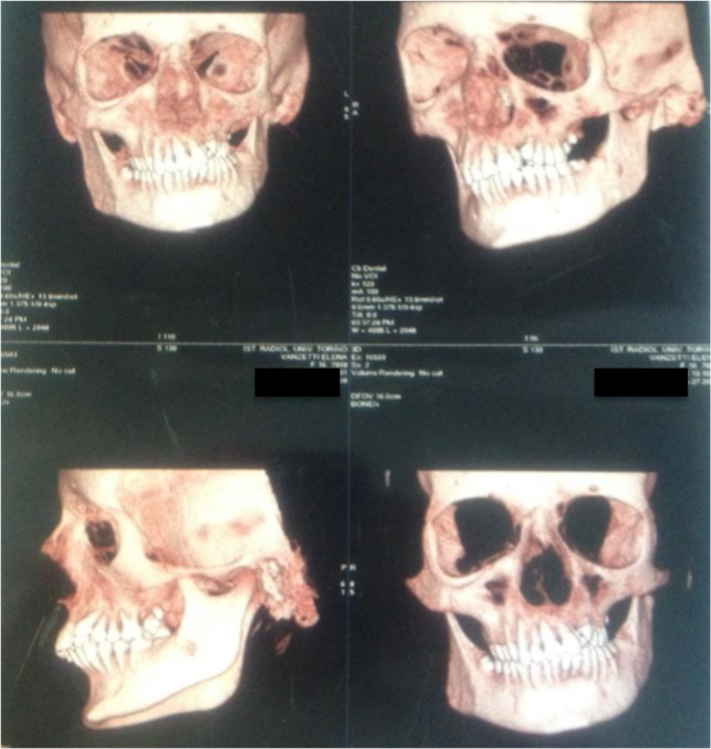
3D CT. 3D CT images show the normal anatomical right and deviated nasal septum, tilt in the occlusal table, hypoplastic zygomatic complex and orbital wall on left side
